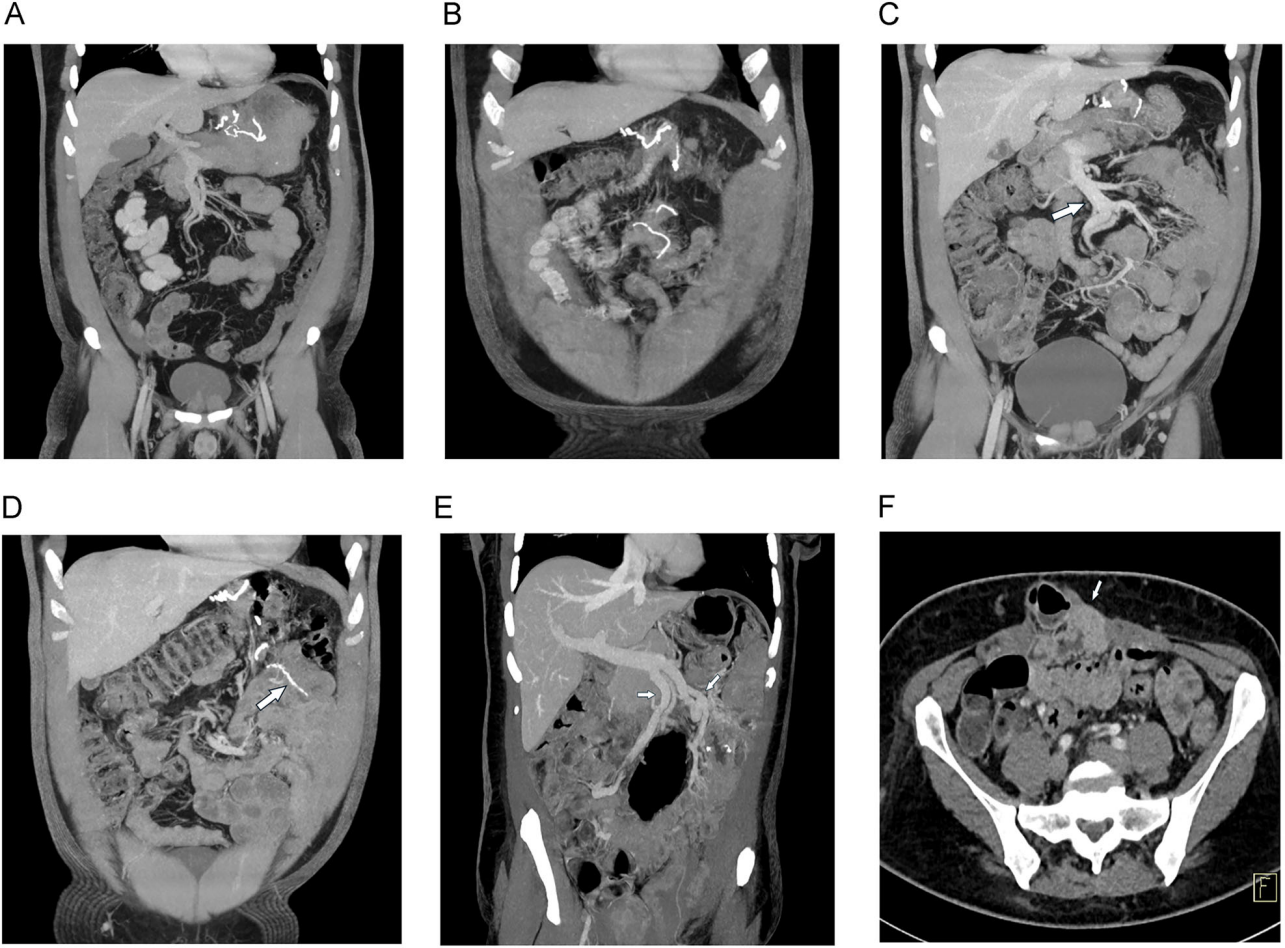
Internal hernia diagnosis during follow-up. A Coronal MIP shows normal course of SMV and (B) normal position of jejuno-jejunostomy (small arrow). Clinical course was uneventful. C, D In a follow-up examination a few months later (due to recurrent abdominal pain), there is (C) new displacement of the SMV and (D) of the anastomosis. Note that neither of the 2 CT studies shows small bowel dilatation. E Pitfall in a patient with a history of small bowel resection and extensive adhesions: There is severe displacement of the SMV (arrows), but no other signs of internal hernia are present. F MDCT also shows an incisional hernia (arrow), which was repaired with a mesh. No internal hernia was found
